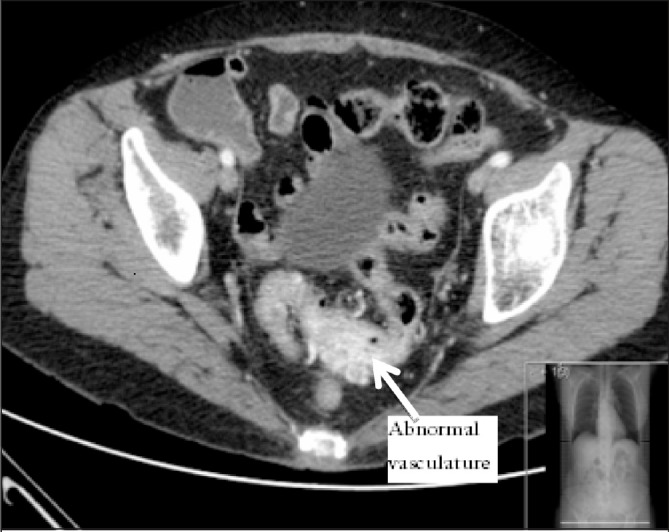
Computed tomography with intravenous contrast; sigmoid thickening demonstrated with abnormal vasculature
